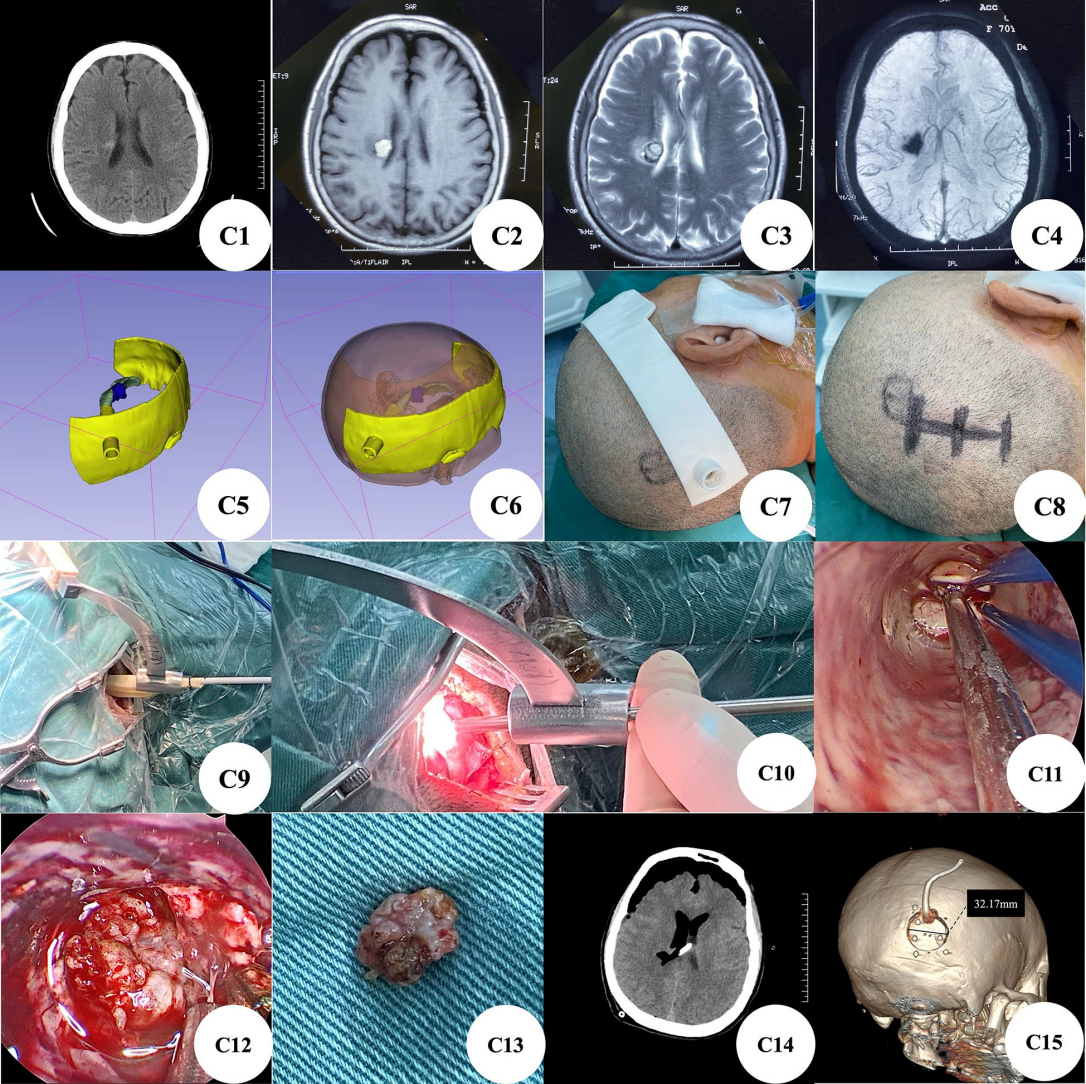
(C1): Preoperative brain CT showed high-density lesions in the proximal ventricle of the right basal ganglia; (C2–C4): Preoperative brain MRI showed lesions in the proximal ventricle of the right basal ganglia; (C5–C6): 3D-Slicer reconstructed the relationship between the lesion and lateral ventricle, and positioned the three-dimensional image of the guide plate; (C7): 3D printing positioning guide; (C8): Straight surgical incision designed according to the printed positioning guide; (C9–C10): Metal 3D guide plate for intraoperative positioning; (C11–C13): Complete resection of lesions assisted by transcranial neuroendoscopic; (C14): Postoperative brain CT showed that the focus was removed completely; (C15): The three-dimensional reconstruction of the skull showed that the size of the bone window was about 3.2 cm.
